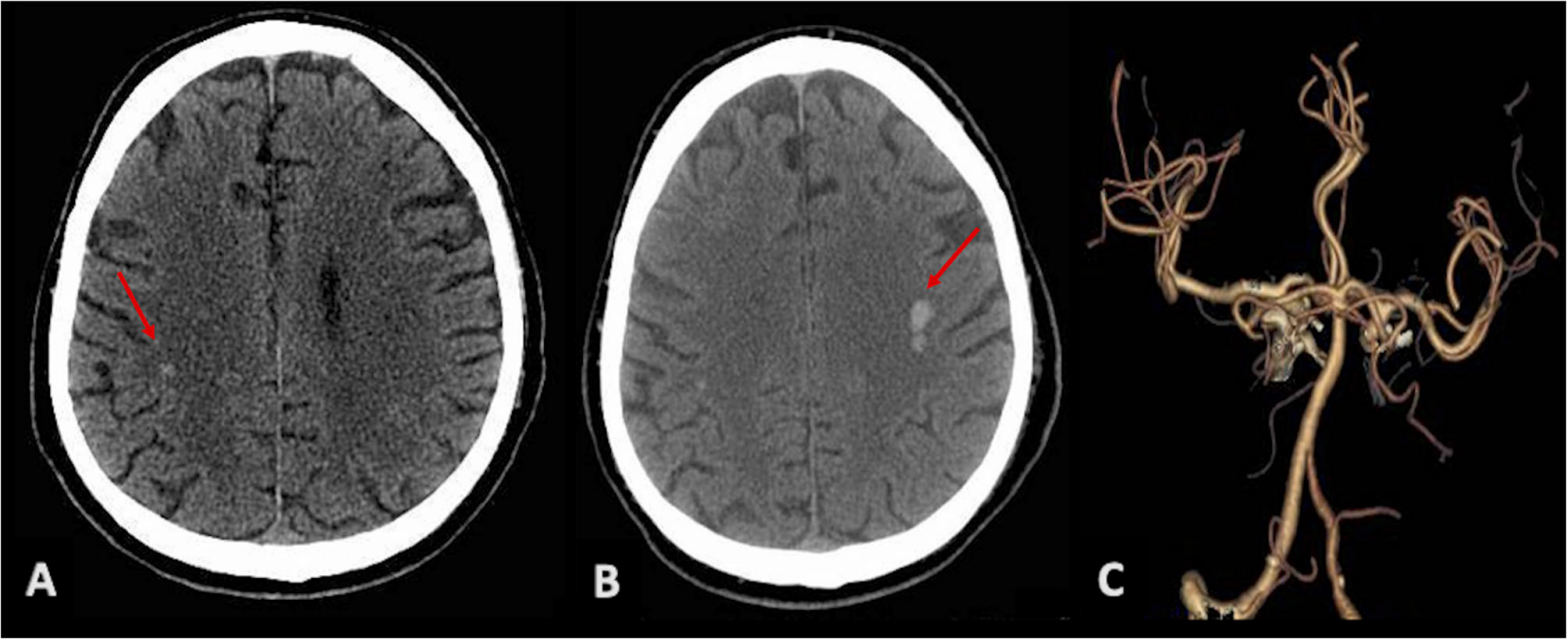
Brain CT scans showing bilateral intraparenchymal hyperdense lesions (red arrows): a small hemorrhagic spot in the post-rolandic area on the right (A) and a greater hematoma in the left pre-rolandic area (B). (C) CTA with no evidence of vascular malformations.
